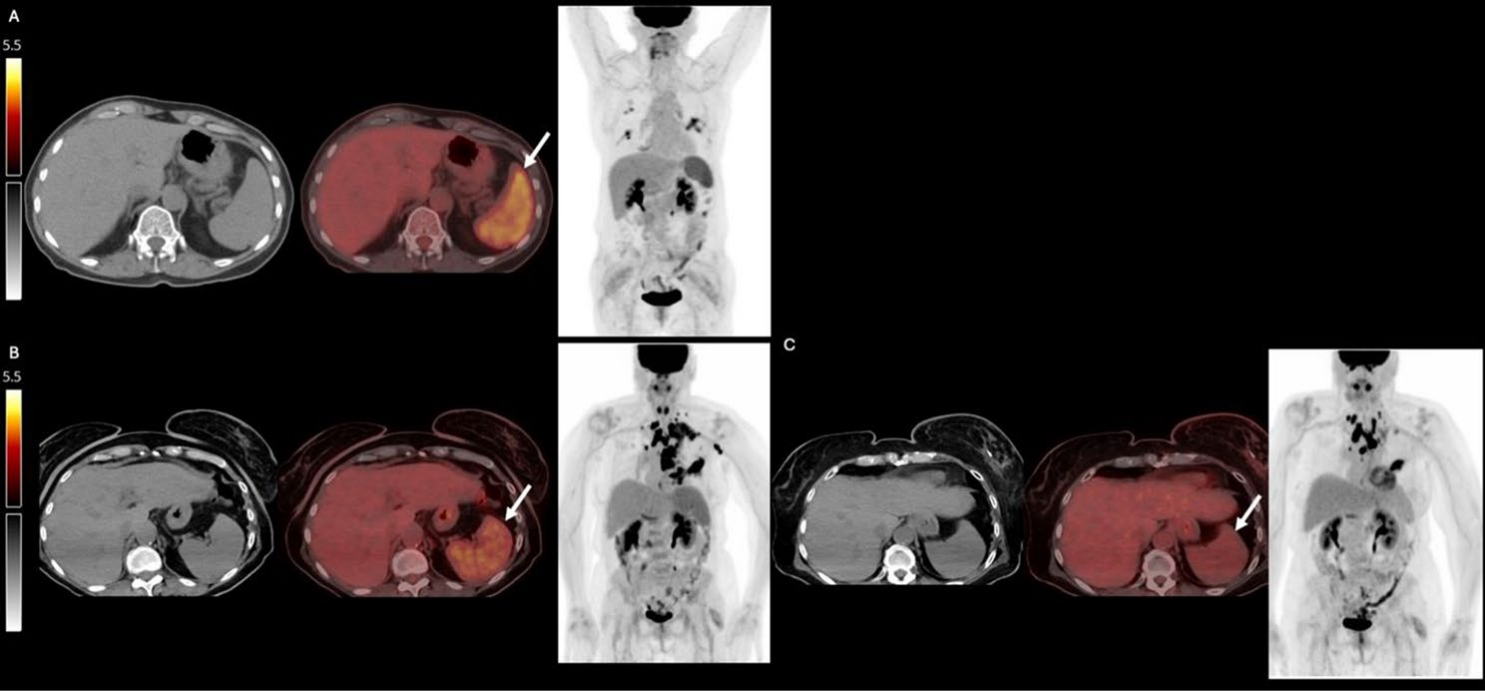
[^18^F] FDG-PET/CT axial CT, axial fused PET/CT, coronal PET maximum-intensity projection images are presented. (A) Patient 1 demonstrates hypermetabolic splenic activity following three months of capivasertib treatment. (B) Patient 2 demonstrates hypermetabolic splenic activity following three months of Capivasertib treatment. (C) Patient 2 demonstrates resolution of hypermetabolic splenic activity after change of regimen from capivasertib to capecitabine
